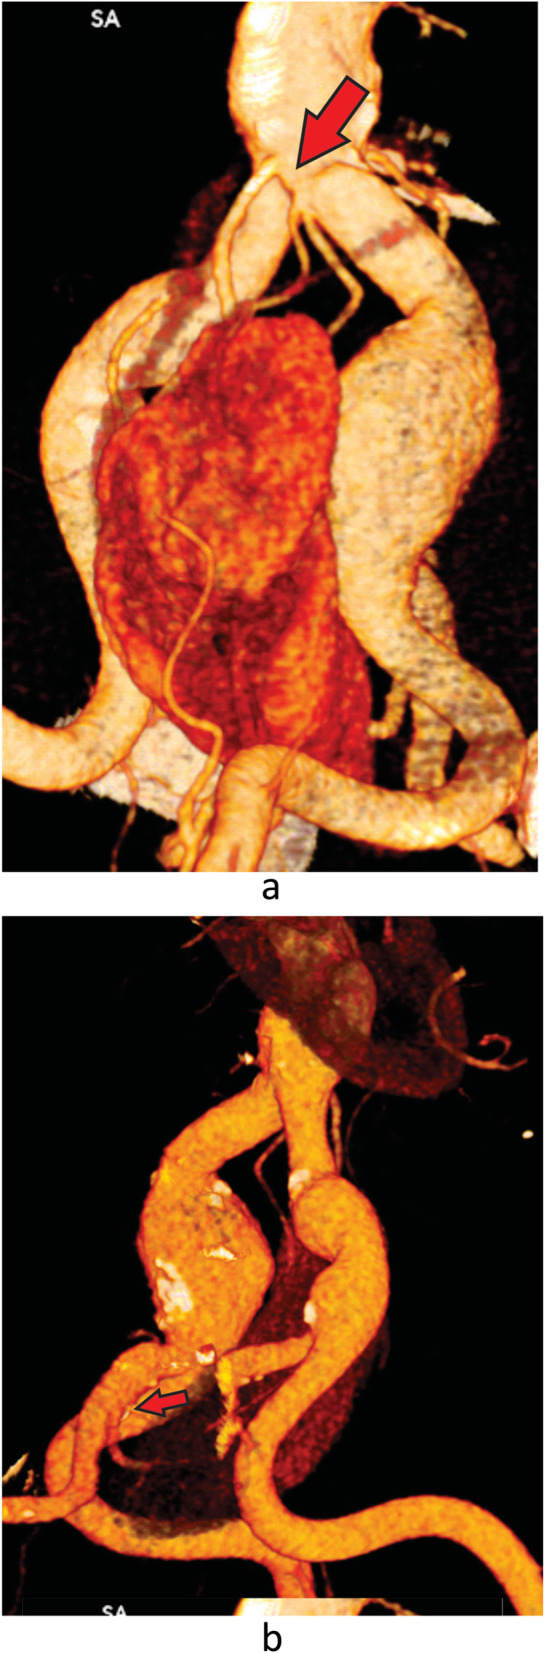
a Reconstructed three-dimensional computed tomography image showing preoperative aneurysm and the pelvic kidney. The polar arteries are visualized. Red arrow. b: Reconstructed three-dimensional computed tomography image showing preoperative aneurysm and the pelvic kidney. The predominant artery that arose from the internal iliac artery are visualized. Red arrow
